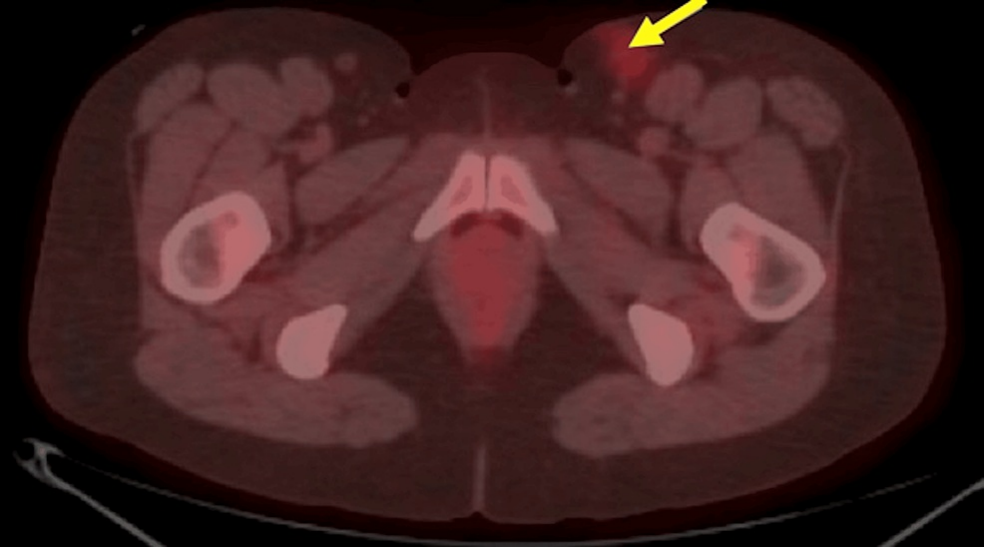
Axial PET/CT imaging demonstrating low-level tracer uptake at the presumed left inguinal excisional biopsy site (blue arrow) and no definite sites of hypermetabolic malignancy. PET/CT: positron emission tomography/computed tomography
